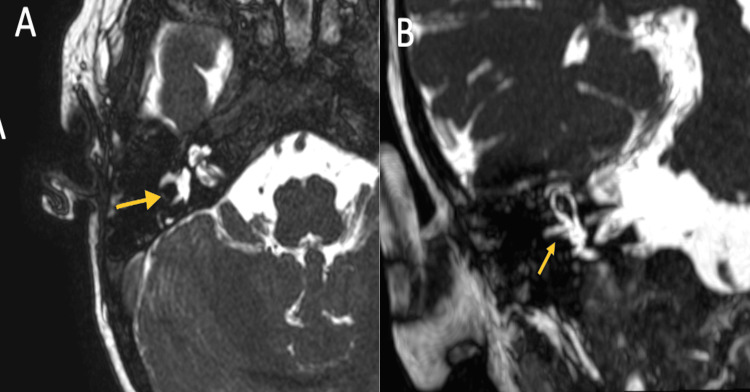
MRI 3D FIESTA sequence on the right side (A: axial plane, B: coronal plane): the hyperintense signal of the lateral semicircular canal ring (yellow arrow) is discontinuous. FIESTA: Fast imaging employing steady-state acquisition
